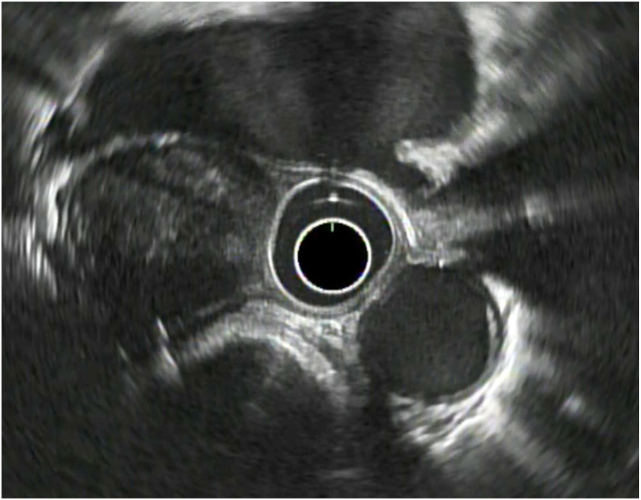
Endoscopic ultrasound of the esophageal lesion. Endoscopic ultrasound demonstrating a well-circumscribed, hypoechoic intramural mass arising from the muscularis propria of the midesophagus, consistent with a subepithelial tumor.
